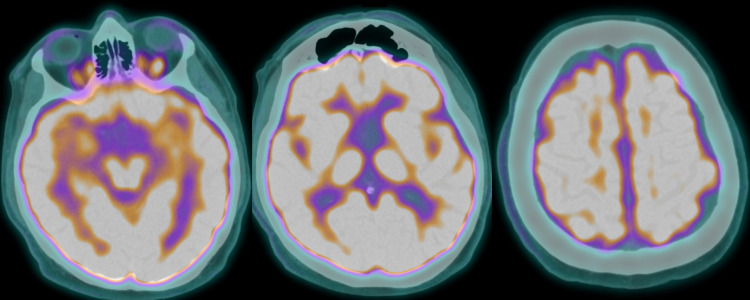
F18-FDG PET/CT scan images displaying no gross diffuse or focal metabolic abnormalities F18-FDG PET/CT: fluorine-18-fluoro-D-glucose Positron Emission Tomography/Computed Tomography
